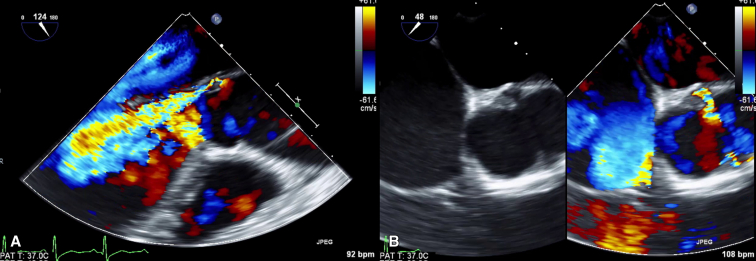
Preoperative transesophageal echocardiography. A, Long-axis color Doppler; B, short-axis color Doppler: paracommissural jets.
